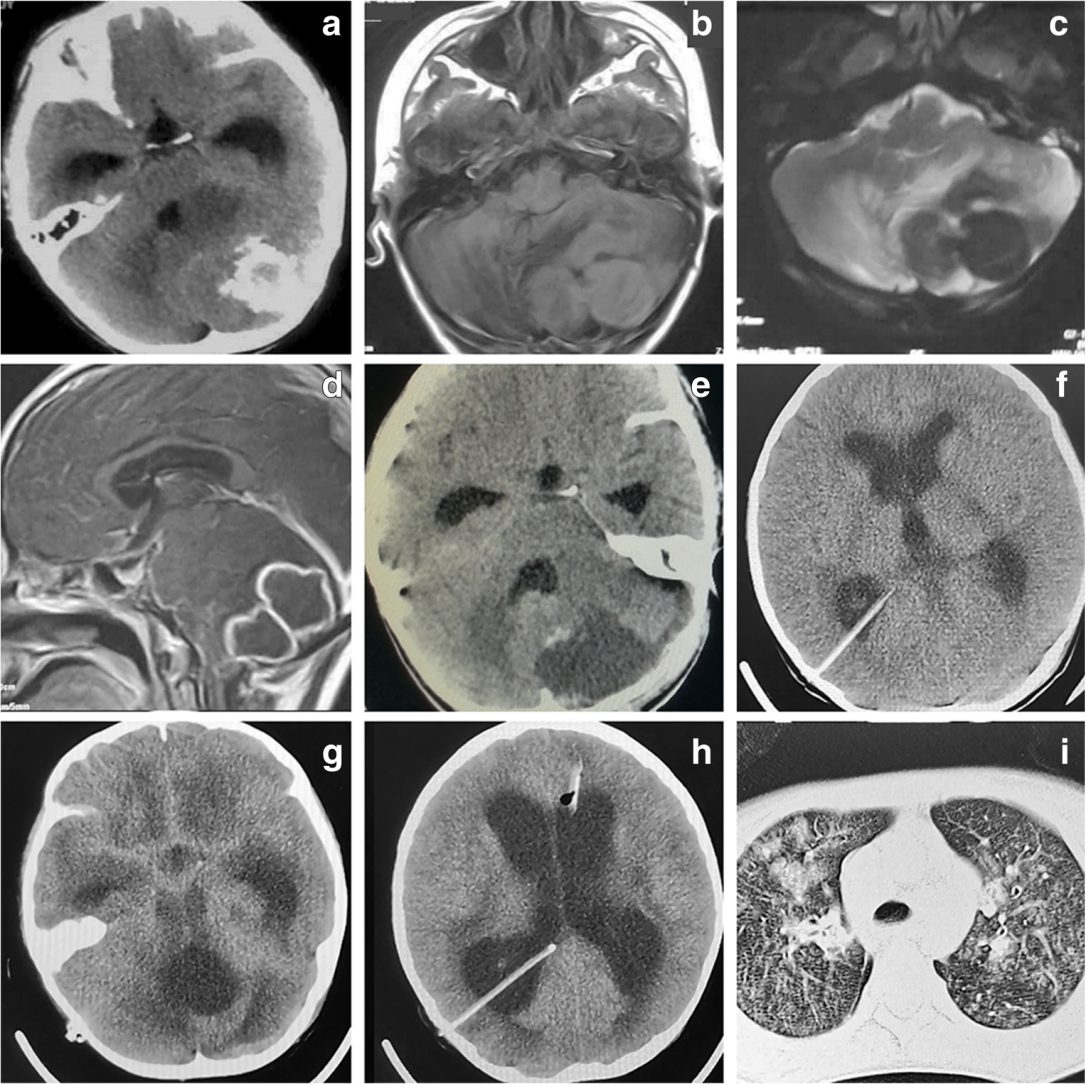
Images of Patient 4 with preoperative EVD. a, CT scan showing big calcified lesion in left cerebellum and hydrocephalus. b, MR T1 showing the lesion is iso-intense and lobulated. c, MR T2 showing low intensity, typical of caseous necrosis and marked peripheral edema. d, MR T1 after gadolinium showing ring shaped enhancement of the lesion and prepontine cistern enhancement, suggestive of meningitis. e, CT scan after lesion resection showing complete removal of the tuberculoma. f, CT scan showing the ventricles shrunk and sulci re-appeared after VPS. g-h, end-stage CT scan showing accumulation of hyperdense pus in major cisterns and still enlarged ventricles after 2nd EVD. i, chest CT scan after deterioration showing lung infection suspected of spreading TB
